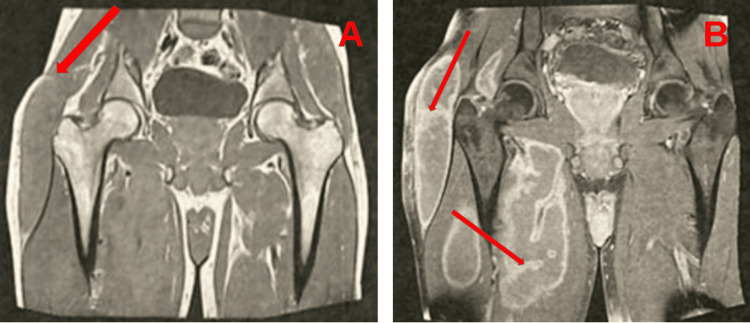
MRI of the left hip (A) Coronal non-contrast image demonstrating a collection arising from the trochanteric bursa (arrow). (B) Coronal contrast-enhanced image showing multiple enhancing parietal villosities (arrows), without signs of locoregional aggressiveness or involvement of adjacent musculotendinous and osseous structures, suggesting hydatidosis.
